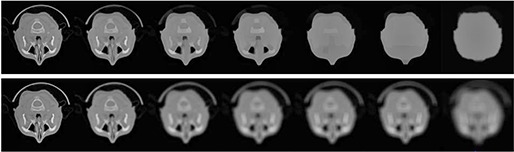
Multiscale decomposition using TV‐L1 scale space filtering in row 1 with λ=0.7,0.45,0.3,0.2,0.15,0.12 and Gaussian scale space filtering in row 2 for CT image with σ=2,4,8,16,32,64, and 80.
